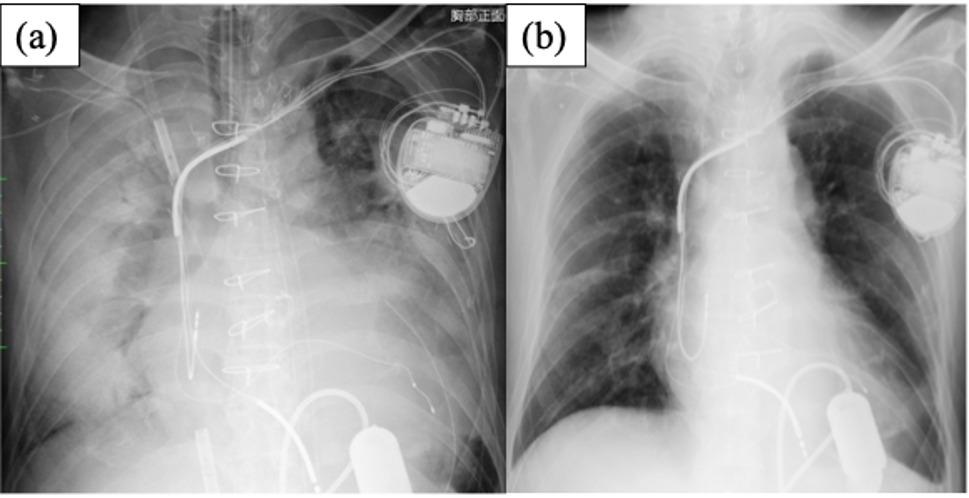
Chest radiographs after Jarvik 2000 implantation. (a) Chest X-ray obtained immediately after initiation of veno-venous extracorporeal membrane oxygenation (VV-ECMO) following Jarvik 2000 implantation for pneumonia-associated acute respiratory distress syndrome. (b) Chest X-ray obtained after V-V ECMO withdrawal. These images demonstrate that severe pulmonary complications were successfully managed with a lung-protective strategy using ECMO, allowing the patient to undergo heart transplantation
